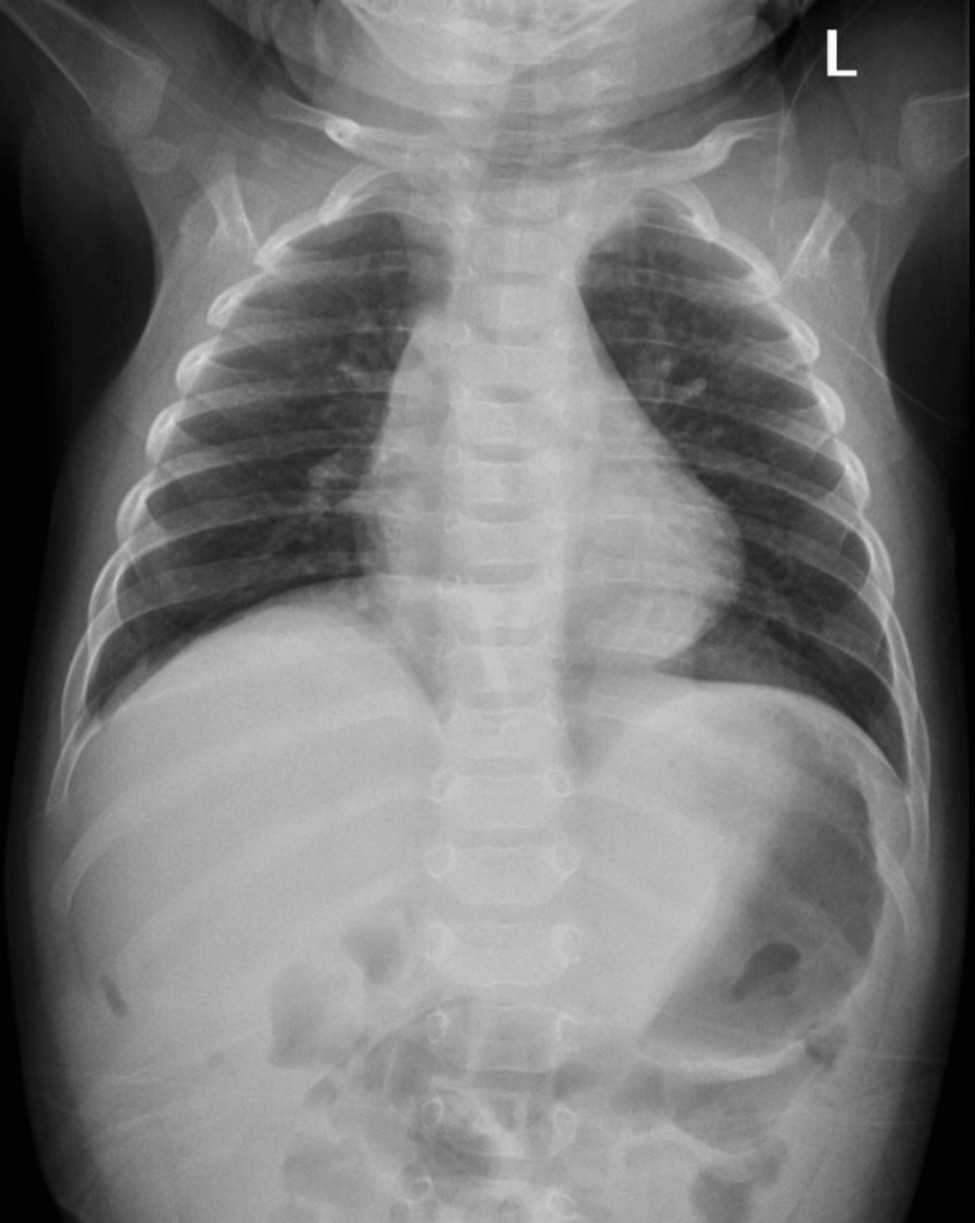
Normal chest radiograph on the seventh day after the surgery
